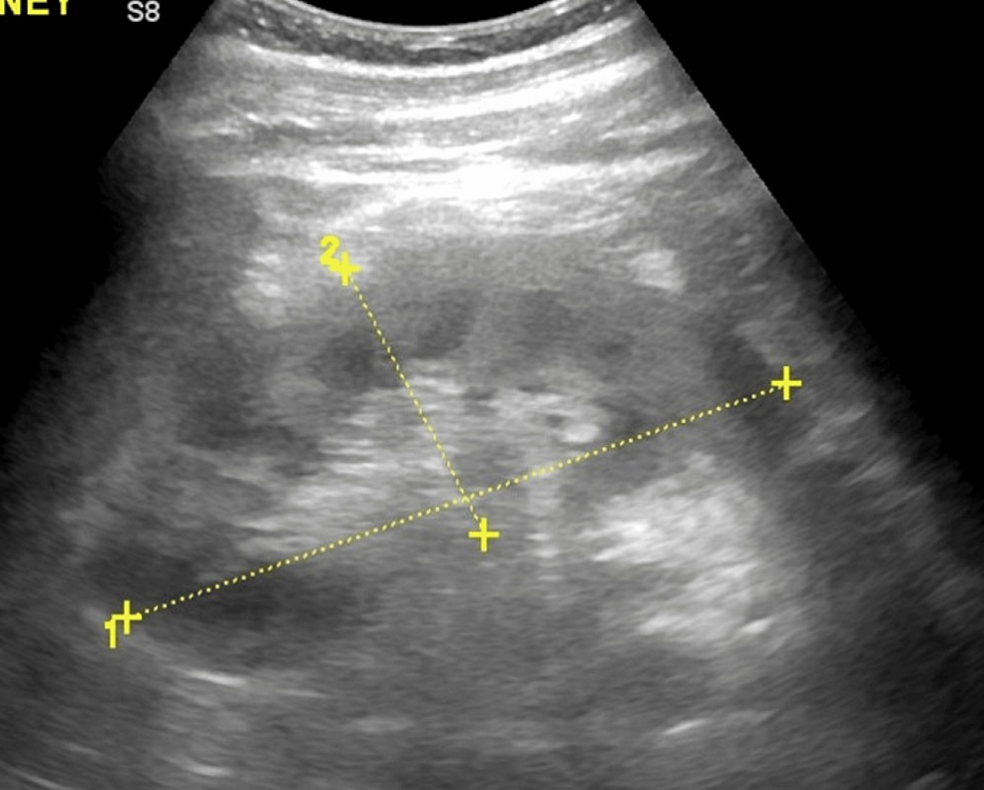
Ultrasound of the left kidney showing a normal kidney measuring approximately 12.4 x 5.3 x 5.7 cm. There were no cysts, solid mass lesions, hydronephrosis, or calculus, and cortical central differentiation was maintained in both kidneys.
